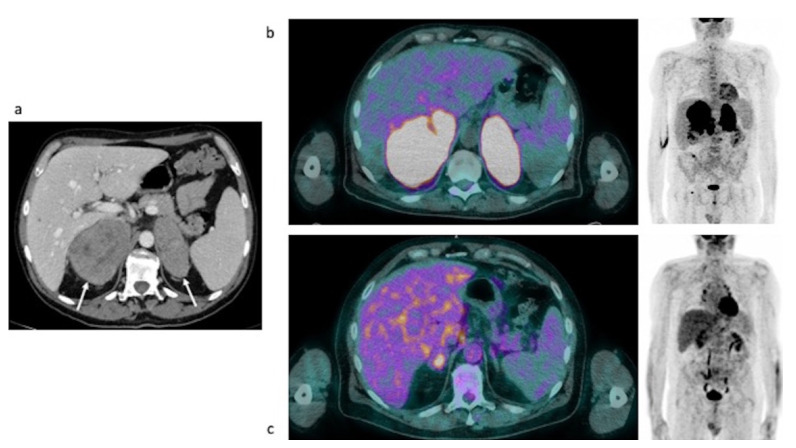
Case #4. Baseline CT (a), baseline and post-therapy FDG PET/CT (b,c).
